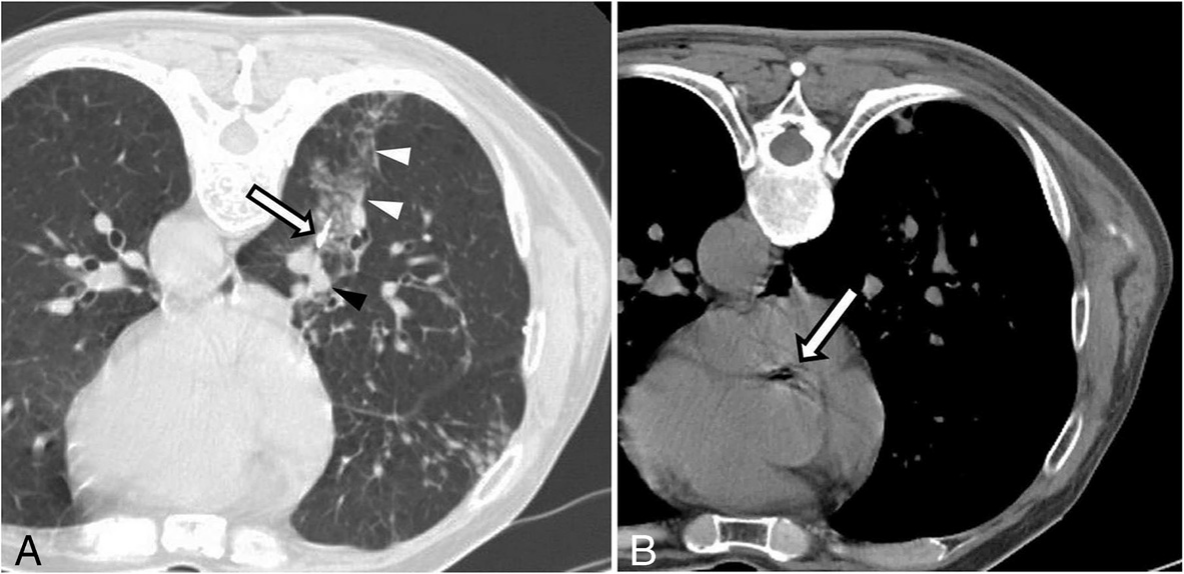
Computer tomography images during and after transthoracic needle biopsy. (A) Prone-positioned CT image obtained during the biopsy shows the biopsy needle (arrow) adjacent to the nodule and parenchymal hemorrhage along the route of the needle (white arrowheads). The pulmonary vein (black arrowhead) is located adjacent to the nodule. (B) CT image obtained immediately after the biopsy shows intraluminal free air in the proximal ascending aorta (arrow).
